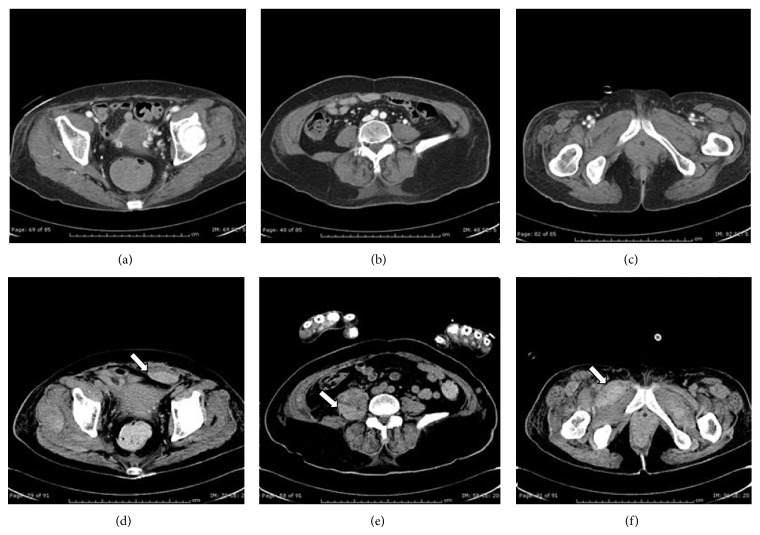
CT scan of the abdomen and pelvis before and after bleeding. (a–c) CT scan with contrast shows (a) normal rectus, (b) normal iliopsoas, and (c) normal pectineus muscles. (d–f) CT scan without contrast shows bleeding into (d) rectus sheath (arrow), (e) iliopsoas (arrow), and (f) pectineus muscles (arrow).
